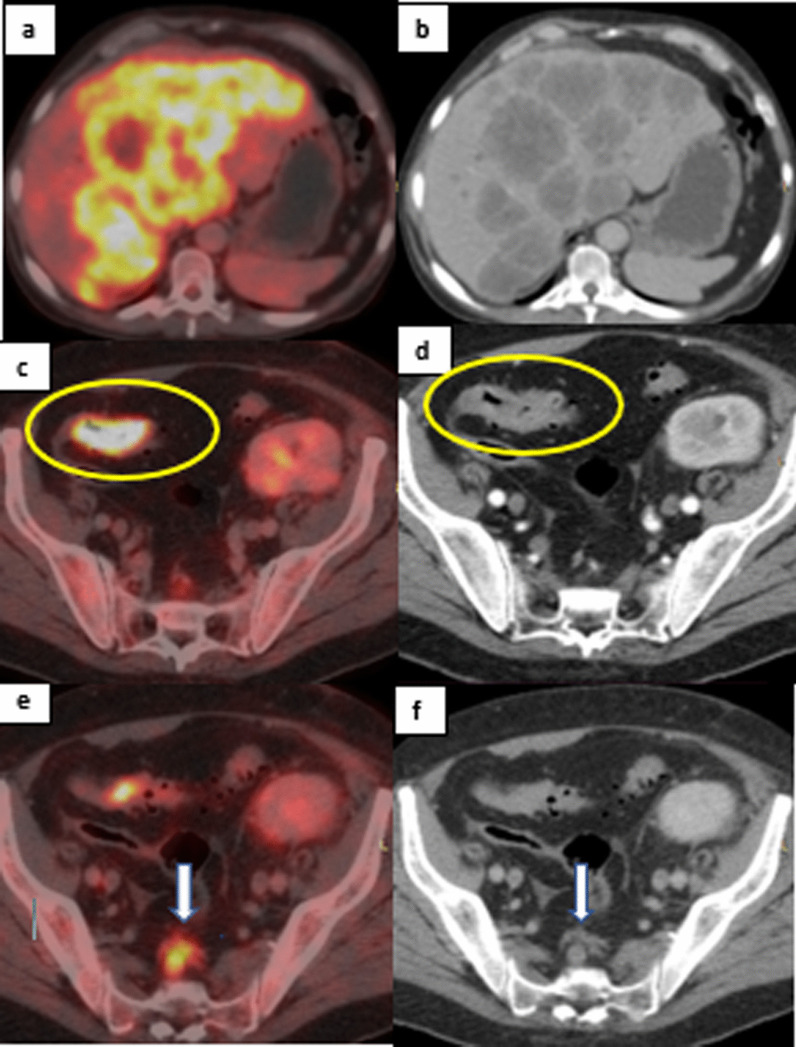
62-year-old male presented with fever of unknown origin with history of renal transplantation. a, b Axial fused PET/CT image of the abdomen and same level axial CT of the abdomen showing large metabolically active hepatic focal lesions with central necrosis. c, d Axial fused PET/CT image and axial CT image of the lower abdomen showing small segment of sigmoid colon FDG avid mural thickening (yellow circles) that showed persistent increased activity in delayed imaging (not shown here). e, f Axial fused PET/CT and CT images of the upper pelvis showing regional enlarged metabolically active presacral lymph nodes (white arrows). Pathology from the sigmoid colon revealed adenocarcinoma
